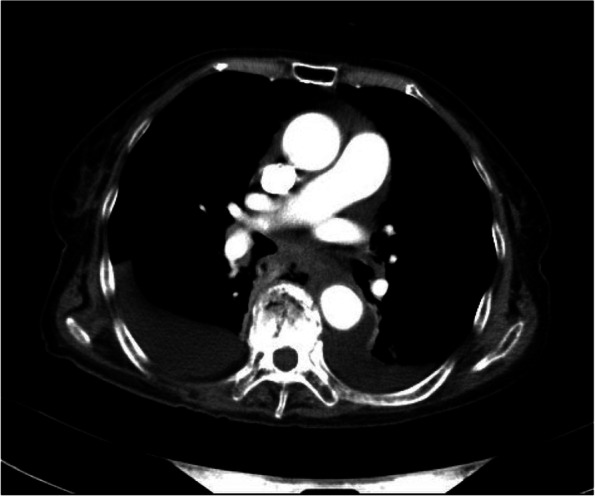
CT scan of the chest shows esophageal-mediastinum fistula
